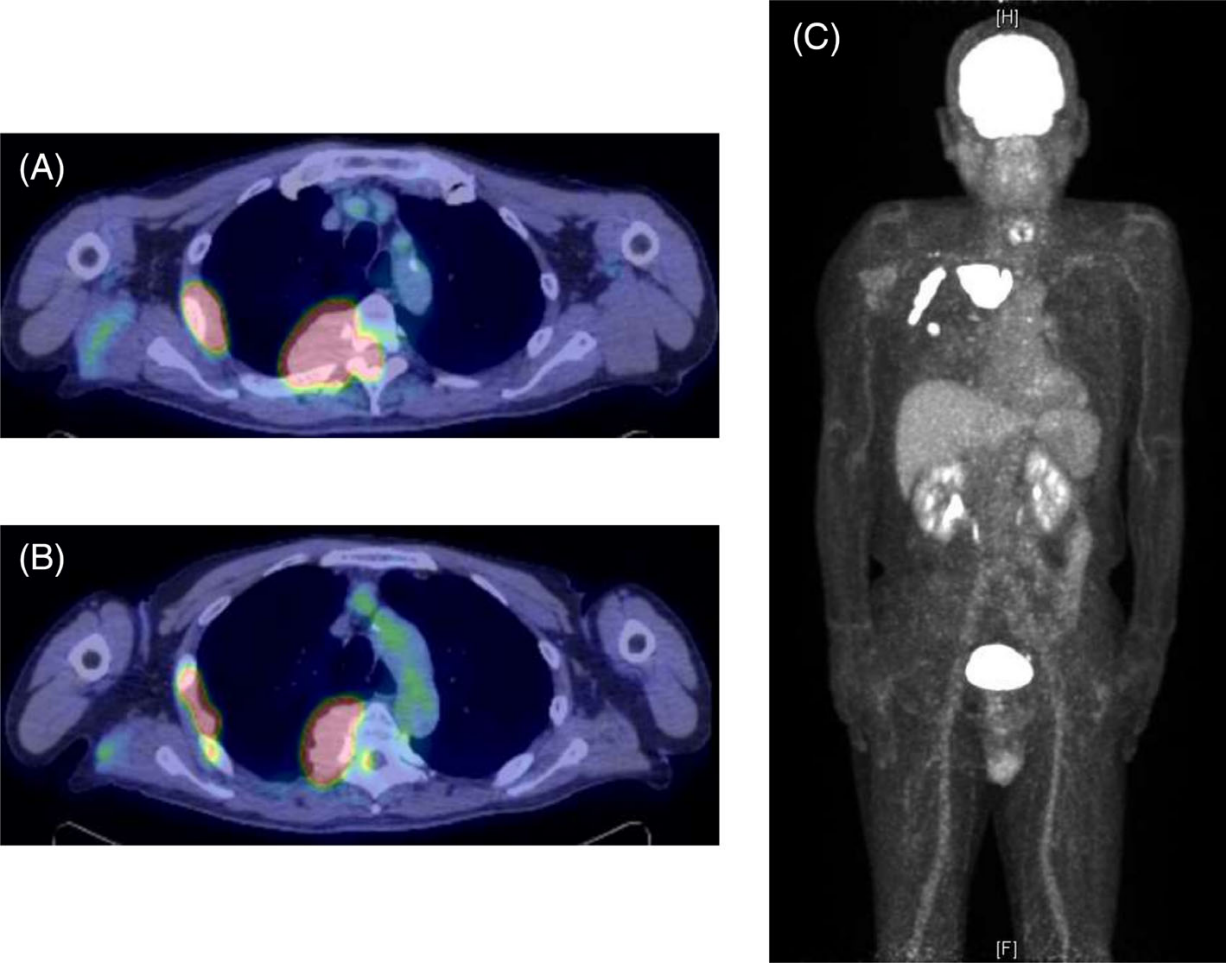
(A–C) Fluorodeoxyglucose‐positron emission tomography (FDG‐PET) scanning revealed high 18‐fluorodeoxyglucose uptake in the right chest wall without any other uptake.
